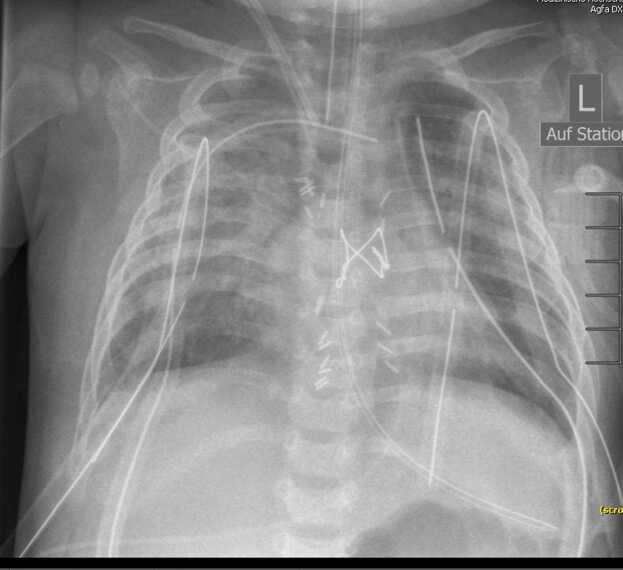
Chest radiograph with ground-glass opacities in a 13-month-old infant with grade 3 PGD after LTX for surfactant protein B mutation.
